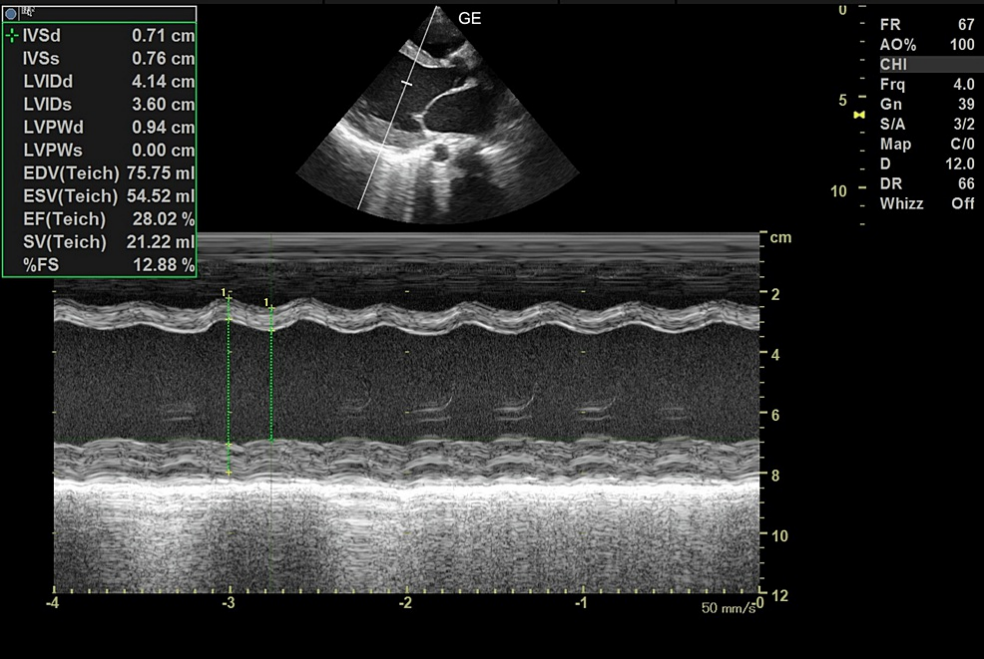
A 2D echocardiogram showing dilated cardiomyopathy with an ejection fraction of 28%.
